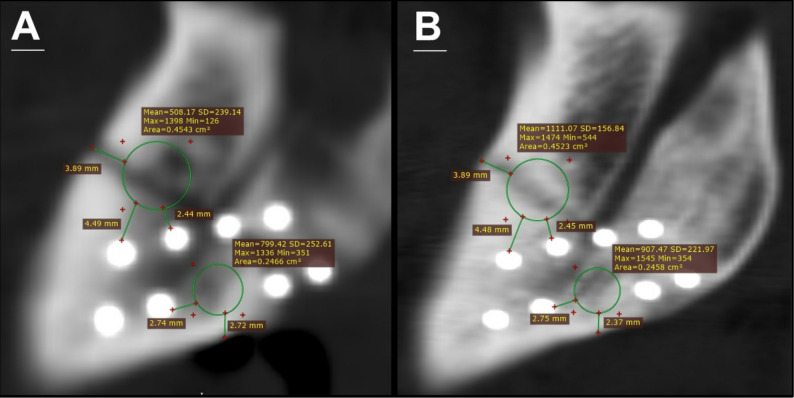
Sagittal view, CT scan analysis of bone density in HU using RadiAnt® DICOM Viewer software. A) Immediate post-operative CT scan analysis of bone density in HU at region of interest delineated over the fracture site. B) 12 weeks follow-up CT scan analysis of bone density in HU at region of interest delineated over the fracture site
